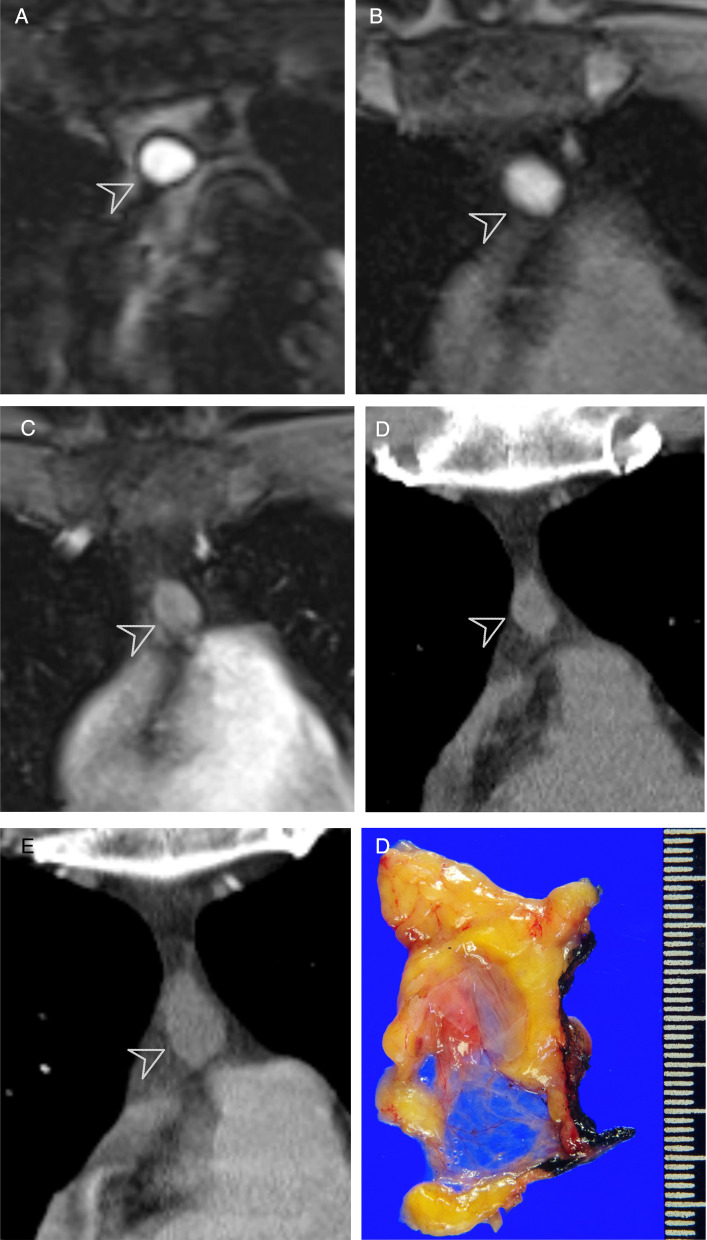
Follow-up outcome of 47-year-old female with incidentally detected anterior mediastinal lesion who underwent chest MRI. On chest MRI, a 19 mm unilocular and ovoid anterior mediastinal lesion shows both high signal intensity on coronal T2 (arrowhead, a) and pre-contrast T1-weighted images (arrowhead, b) and no remarkable enhancement on post-contrast T1-weighted image (arrowhead, c). Patient underwent annual follow-up for the anterior mediastinal lesion. Compared to the baseline non-contrast coronal chest CT image (arrowhead, d), the lesion size increase from 15 to 25 mm on follow-up coronal contrast-enhanced chest CT image after 3 years (arrowhead, e). Patient underwent thymectomy, and the gross pathology shows a 25-mm well-defined ovoid cyst with cystic content of mucinous material (f). The pathologic diagnosis was a thymic cyst
